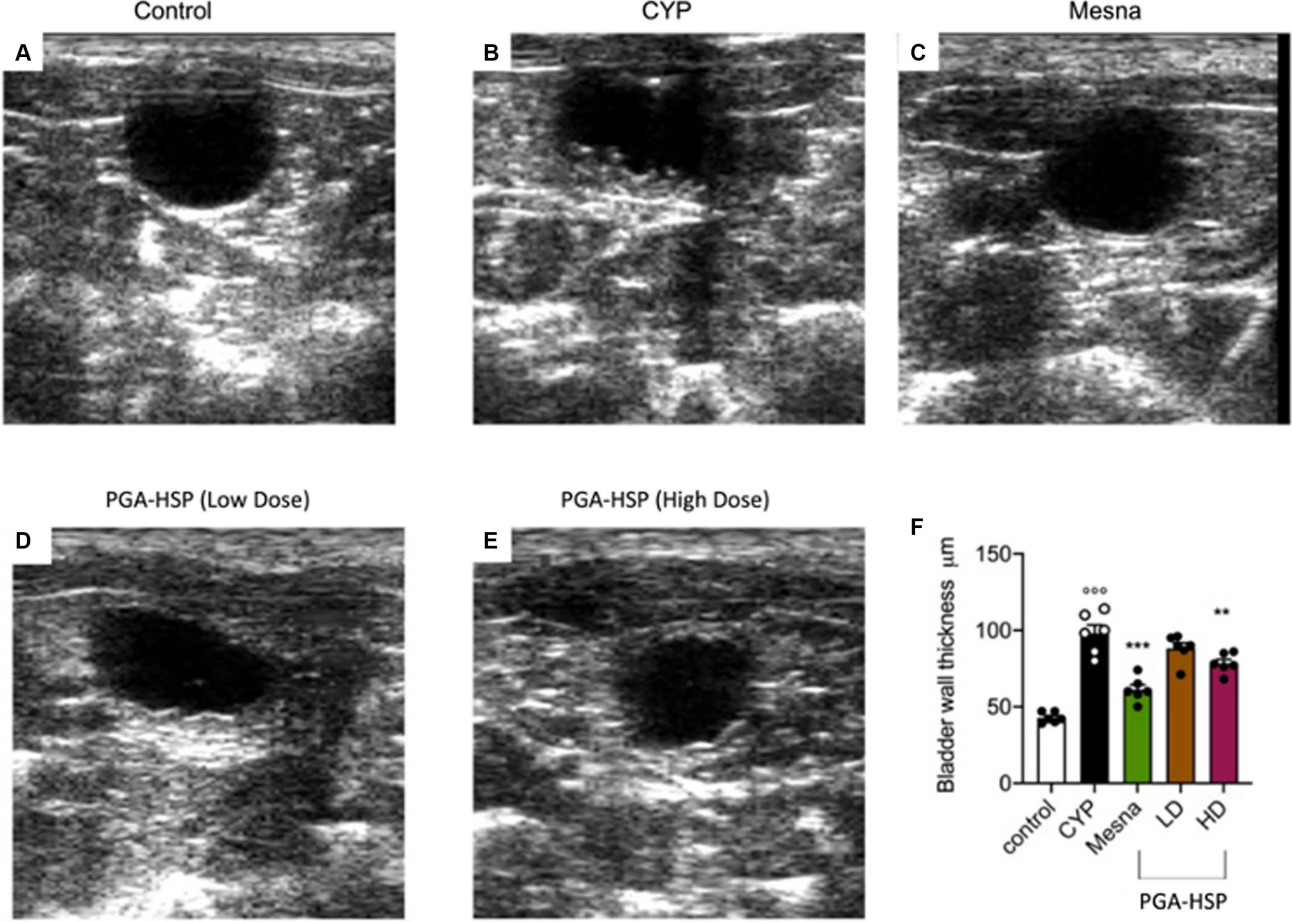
Ultrasound longitudinal view images of the urinary bladder in the different treatment groups, moderately distended with anechoic saline solution (A–E). Bladder wall thickness in the different treatment groups is reported in the histogram (F). Data are expressed as mean ± SEM (*n* = 6), figures are representative of three independent experiments. CYP, Cyclophosphamide; HD, high dose; LD, low dose; PGA-HSP, 3:1 mixture of micronized palmitoyl-glucosamine and hesperidin. *p* < 0.01 versus CYP; p* < 0.001 versus CYP; °°°*p* < 0.001 versus control.
